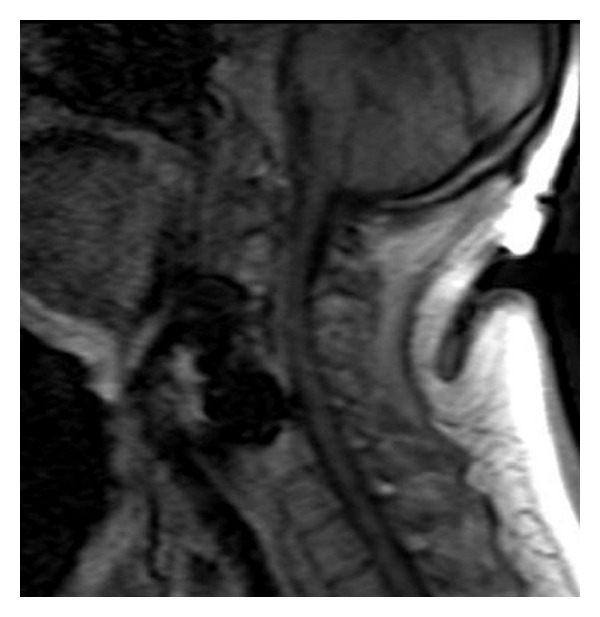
Postoperative MRI in sagittal plane confirming the drainage of the abscess and the subsequent release of the spinal cord pressure.
